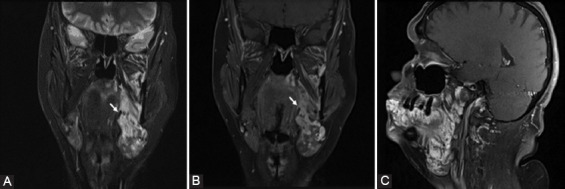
A 51-year-old woman with a lump in the right submandibular region confirmed by magnetic resonance imaging to be a venous malformation. (A) Coronal short-tau inversion recovery image demonstrates the presence of heterogeneous lesions, predominantly showing high-intensity signal at the level of the entire hemi-face on the left, involving superficial and profound tissues as well. (B) Coronal and (C) sagittal gadolinium-enhanced fat-suppressed T1WI demonstrates the presence of multiple serpiginous vessels. Phleboliths may be observed (arrow).
